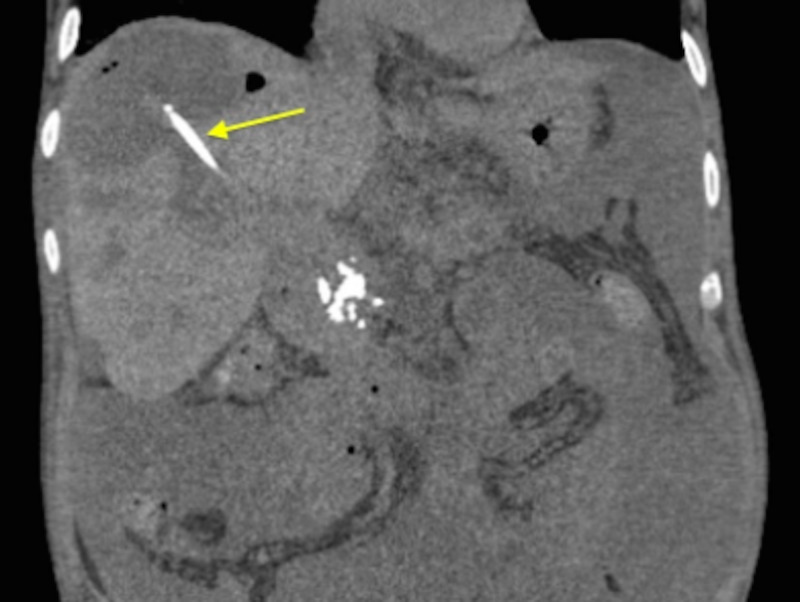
Post-procedural CT of the abdomen with an arrow demonstrating the stent within the biloma
